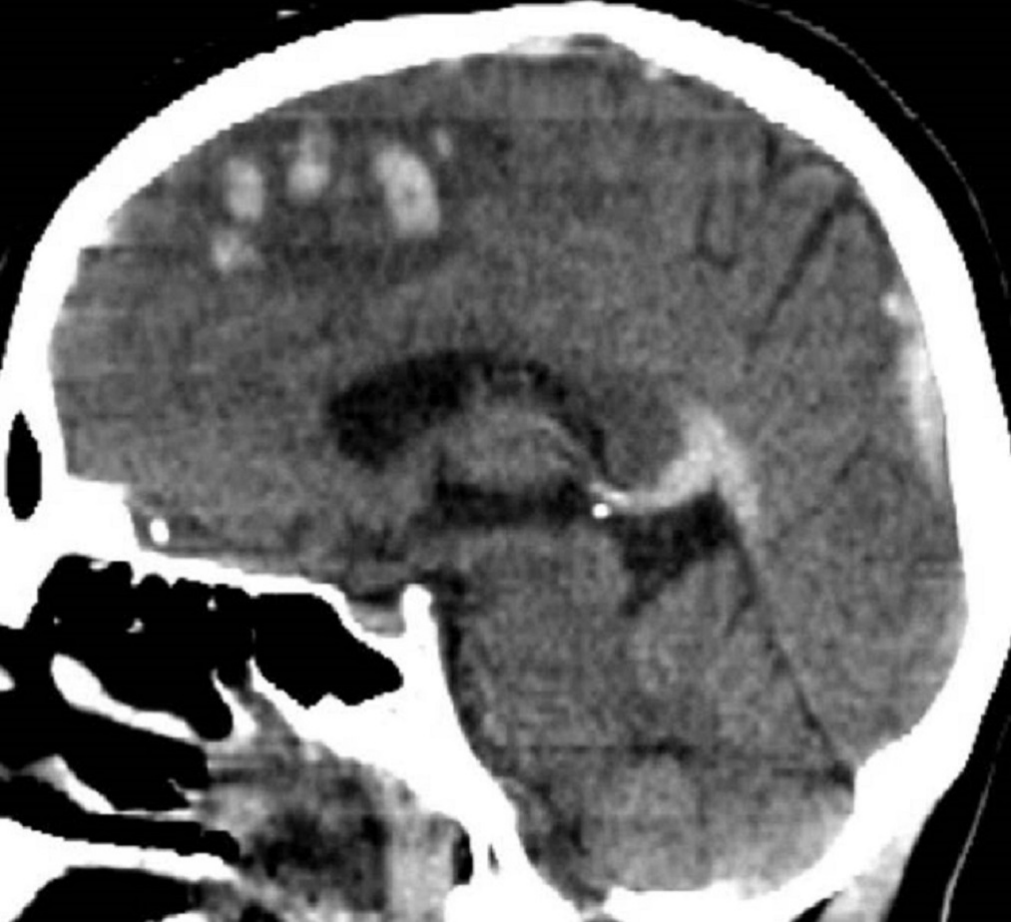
NON-Contrasted CT at Presentation NON-contrasted CT obtained at presentation in the sagittal view reveals hyperdense thrombus in the sagittal sinus, vein of galen, and the straight sinus. A classic appearing venous infarct with hemorrhage and very little mass effect are seen.
